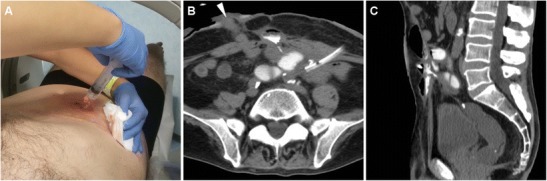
CT-fistulography technique, directly performed on the scanner table by manual injection through the EFO of diluted CM using a syringe and a venous cannula (A). An example of CT-fistulography (B, C) in a 69-year-old man with ulcerative colitis, previous right hemicolectomy and repeated surgery for complications: shortly after ileostomy closure (note drainage tube) low-output ECF was diagnosed, with communication between the EFO (arrowhead in B) and the opacified ileum
